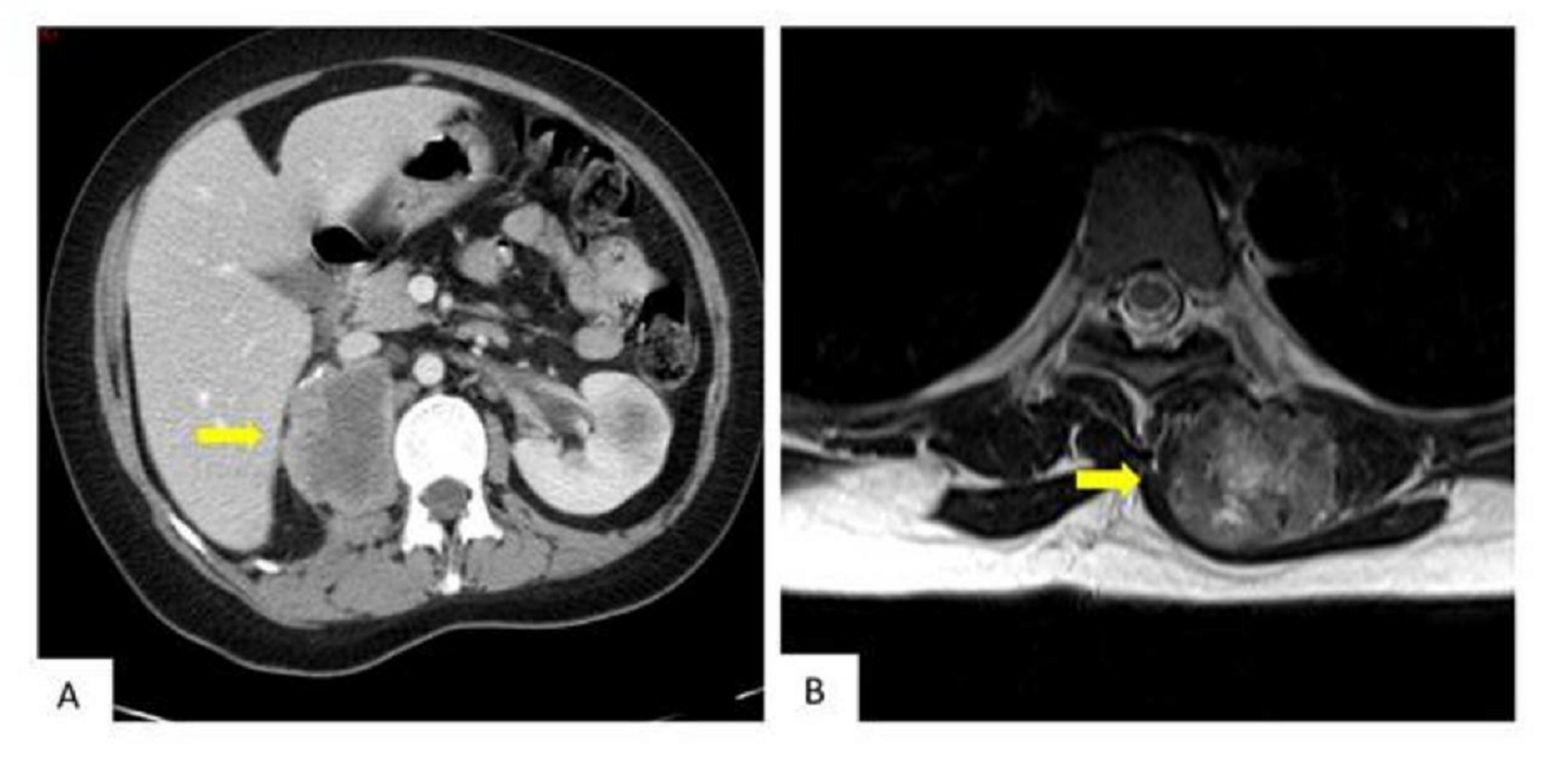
Follow-up Images (Abdominal and Pelvic CT Scan/MRI) A: Computed tomography (CT) of the abdomen in axial section six months after the initial, showing tumor relapse in the right nephrectomy bed and new left renal lesion similar to that documented in the right kidney. B: Magnetic resonance imaging (MRI) in T2 sequence showing the left paraspinal lesion in progression.
